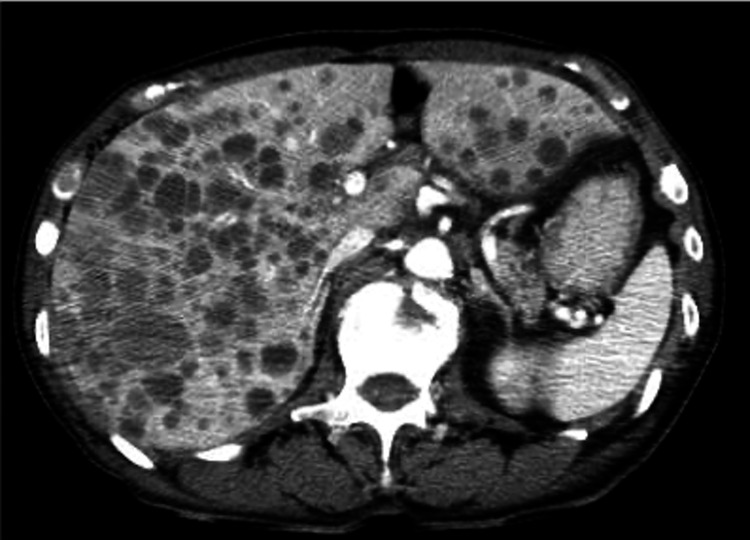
Imaging finding at disease progression CT image showing multiple liver metastases. CT: computed tomography
